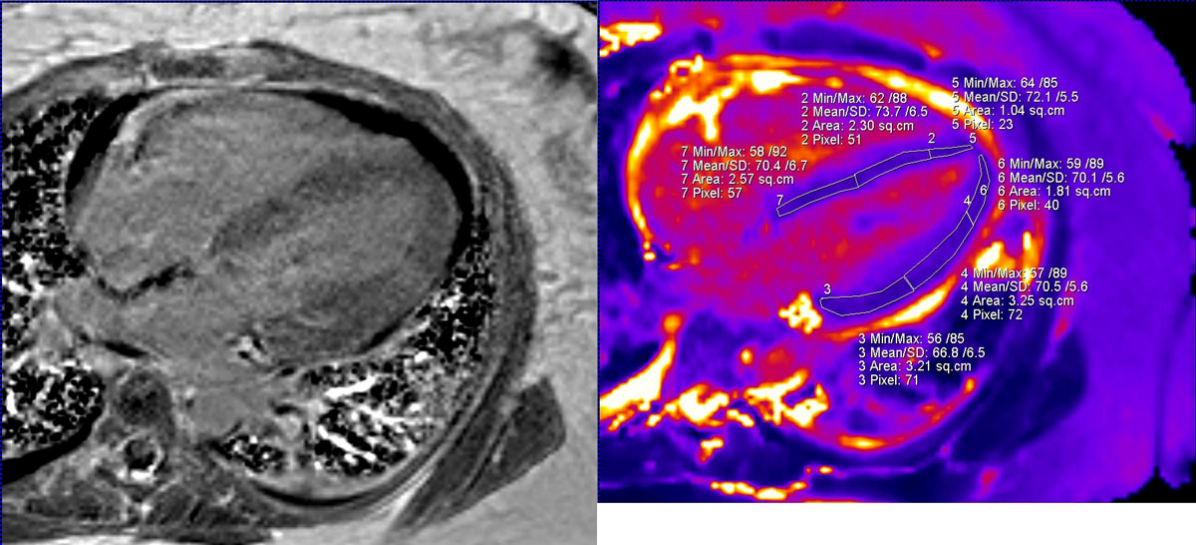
4 Chamber views. IR LGE image (left) showing a small patchy area of LGE with no other area of obvious LGE. T2 map (right) shows elevated T2 values in all the visualized myocardial segments. This patients troponin on the day of MRI was 1.26 with the EF of 39%.
